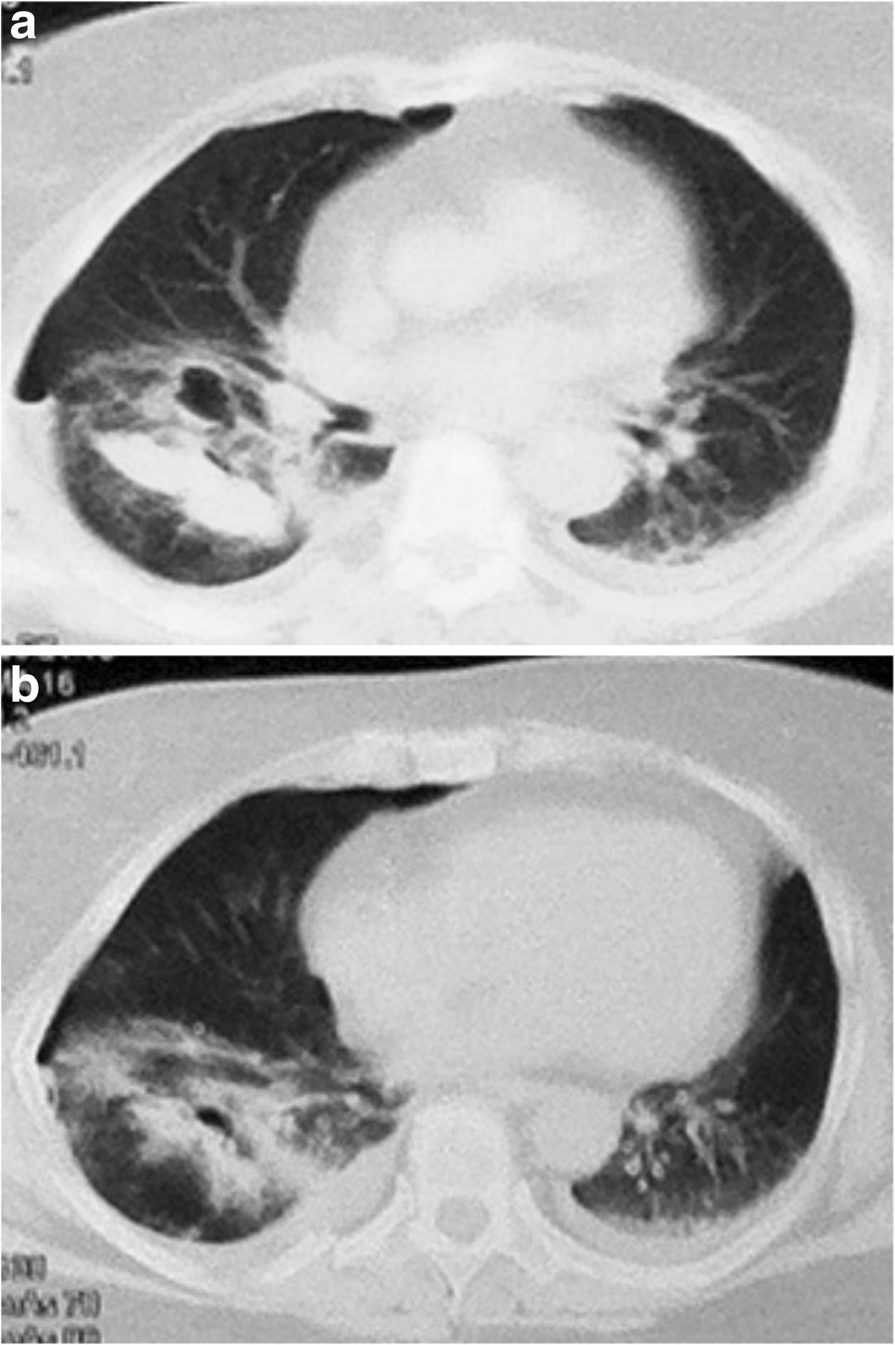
Axial computed tomography of patient’s blunt chest trauma. (A) Axial computed tomography shows a low-percentage pneumothorax and a thin-walled air cavity on the anterior segment of the right lower lobe in close contact with the interlobar fissure. (B) Axial computed tomography shows an extensive contusion in the posterior and lateral segments of the right lower lobe, and the presence of air is demonstrated.
